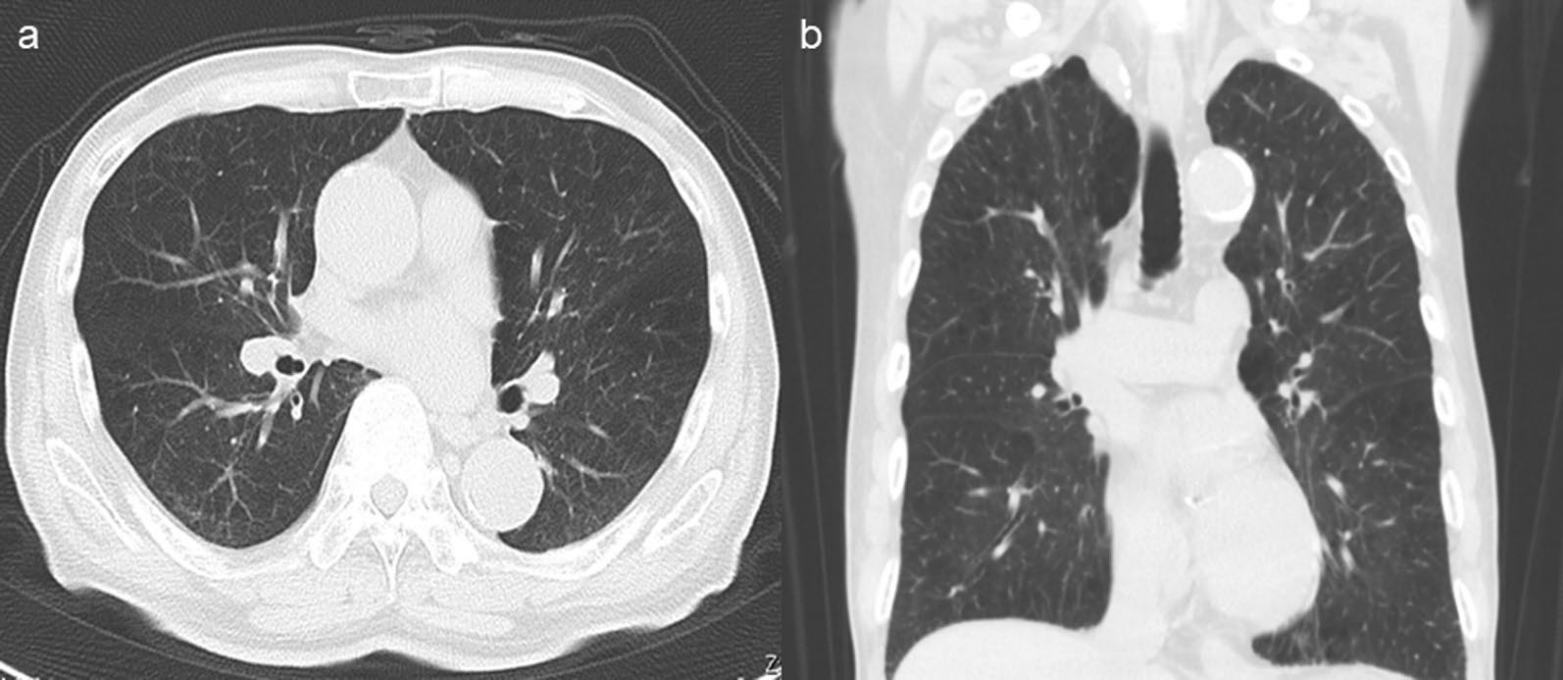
Chest computed tomography. There were no findings of pneumonia. a Axial view. b Coronal view
